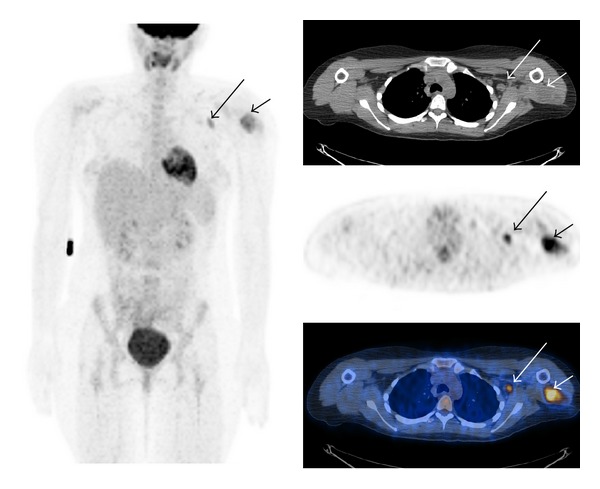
False Positive a 29 year old with an angiomatoid malignant histiocytoma of the left deltoid (short arrow) with an SUV of 6.5. The FDG-PET scan demonstrated a hypermetabolic left axillary lymph node (long arrow) with an SUV of 4.4. The latter was biopsied and was a reactive lymph node on final pathology.
